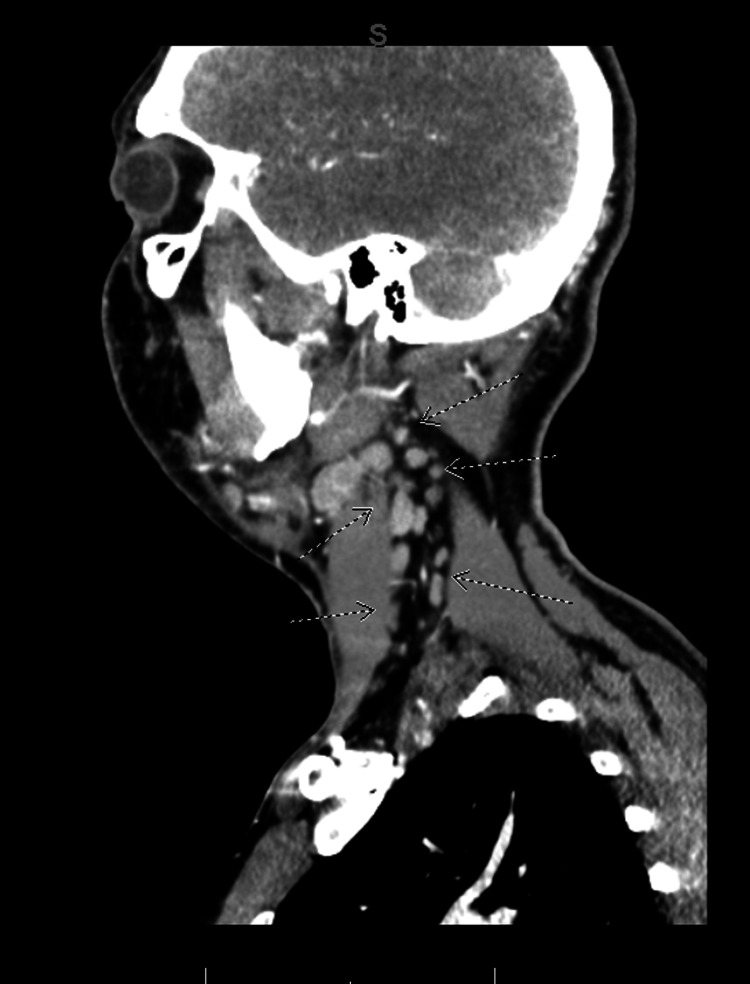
Initial sagittal contrast-enhanced CT with arrows indicating areas of soft tissue edema and reactive lymphadenopathy secondary to pharyngitis.
